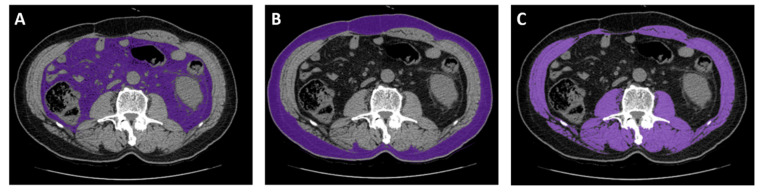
Representative computed tomography measurements of tissues using analysis software. (A) Visceral adipose tissue. (B) Subcutaneous adipose tissue. (C) Skeletal muscle.
